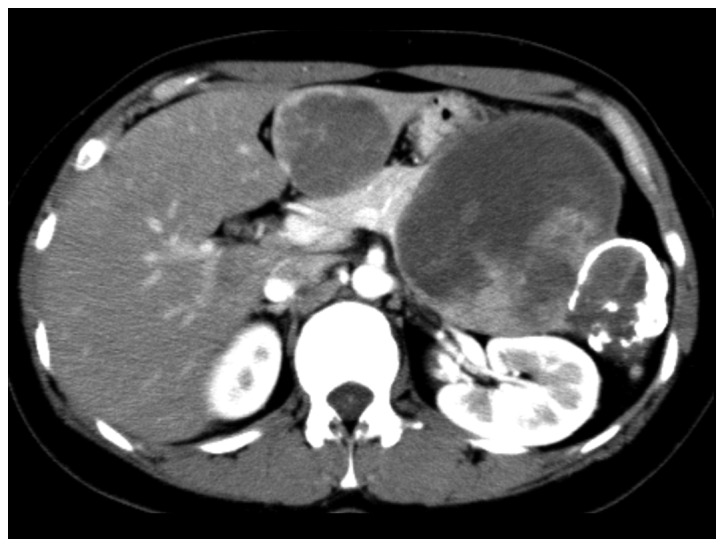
Abdominal computed tomography image following previous hospital admission. A cystic tumor of 10 cm in diameter in the pancreatic tail (with calcification) and multiple liver tumors were detected.
